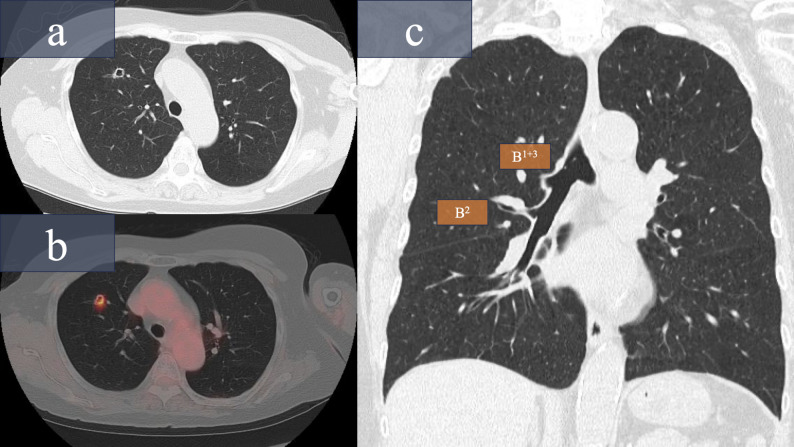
Preoperative imaging findings. a Computed tomography (CT) showing a 12-mm partially solid nodule with a cavity in the right upper lobe at the border between segments S1 and S3. b
